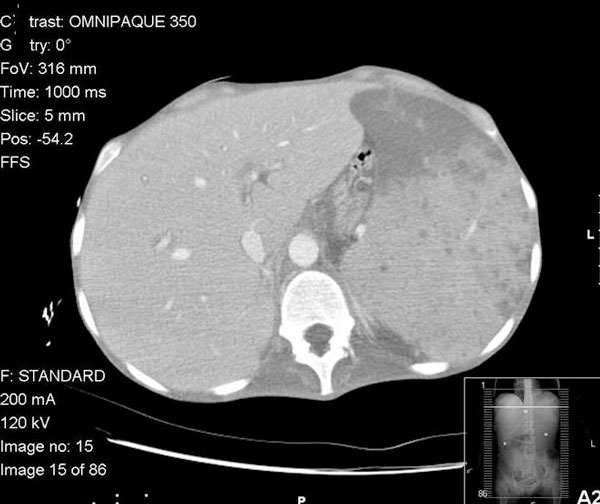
Abdominal CT scan with intravenous contrast showing hepatomegaly and enlarged spleen with multiple hypo dense lesions.
